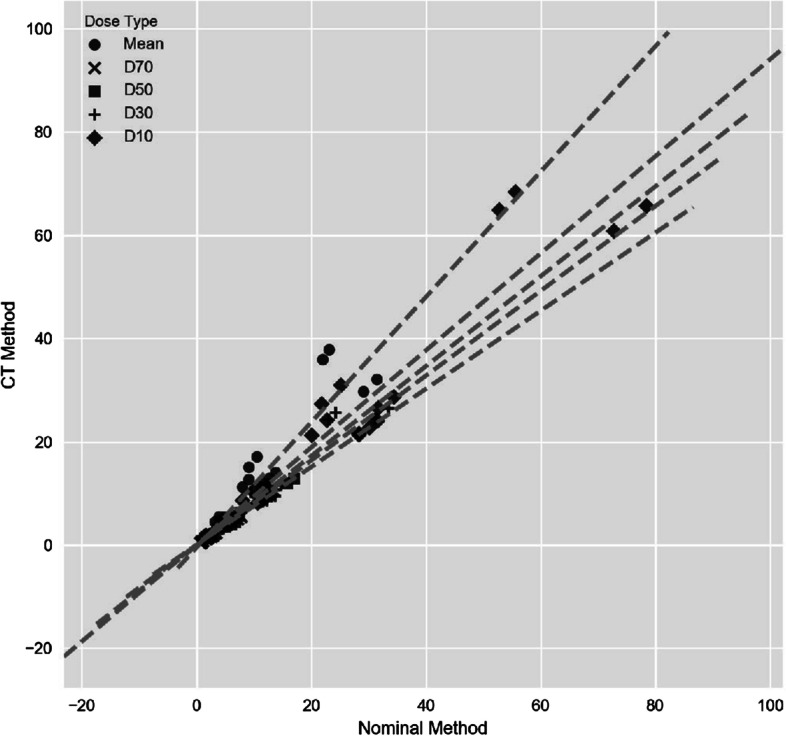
The linear regressions represent weighted dose factors that compares the mass density differences between the CT and nominal local deposition methods for a particular dose metric, $$N=20$$. *N* represents the number of data points compared for each dose metric for the lungs
